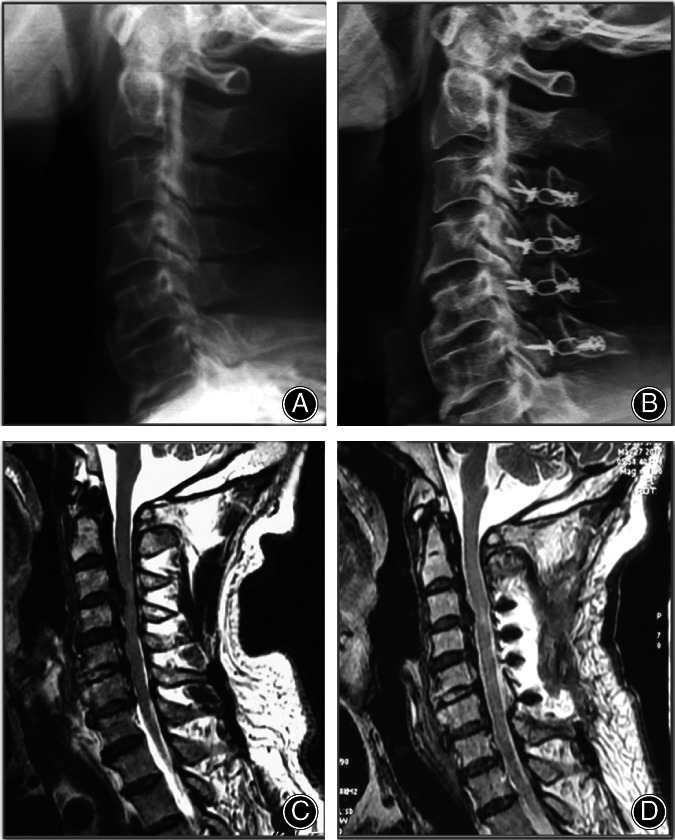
MRI and X‐ray scans showing the shifting of spinal cord after the ultrasonic curette‐assisted laminoplasty. Figure A and C showing the radiograph and MRI sagittal view of the cervical spine before the surgery. B and D showing the radiograph and MRI sagittal view of the cervical spine after the surgery. Note that the spinal cord was loosened in the lower part of C_2_ level.
